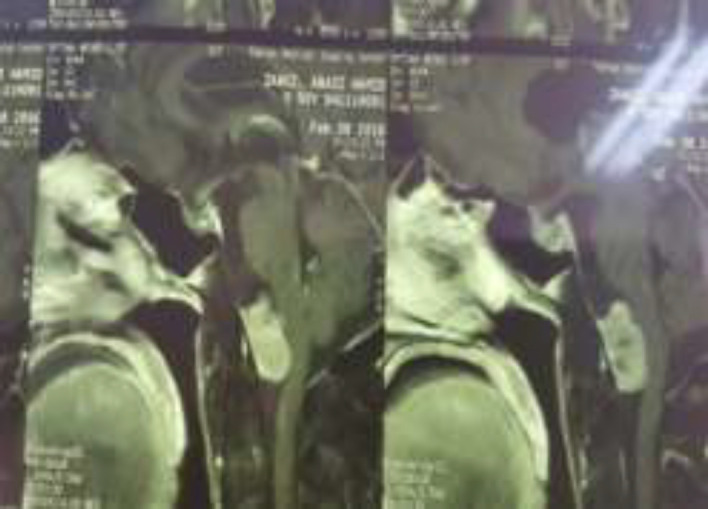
Preoperative T1 imaging (MRI) shows enhancing mass in the craniocervical junction (Ant cistern of brain stem) with compressive effect on ant medulla
